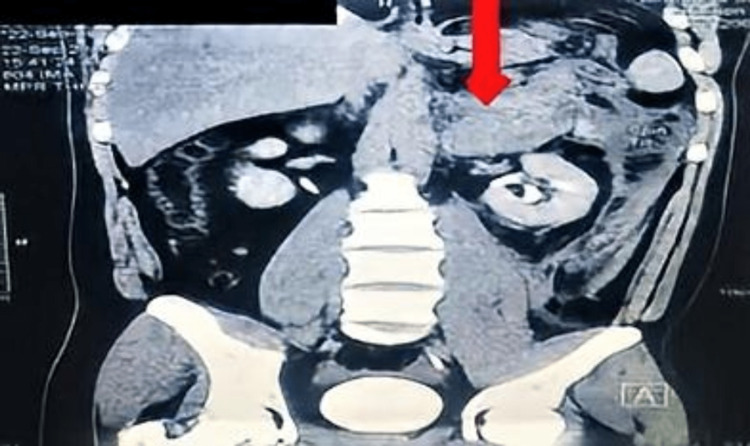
Multiphasic CT of adrenal glands coronal section showing a left adrenal heterogenous mass pushing the left kidney downwards (arrow).
